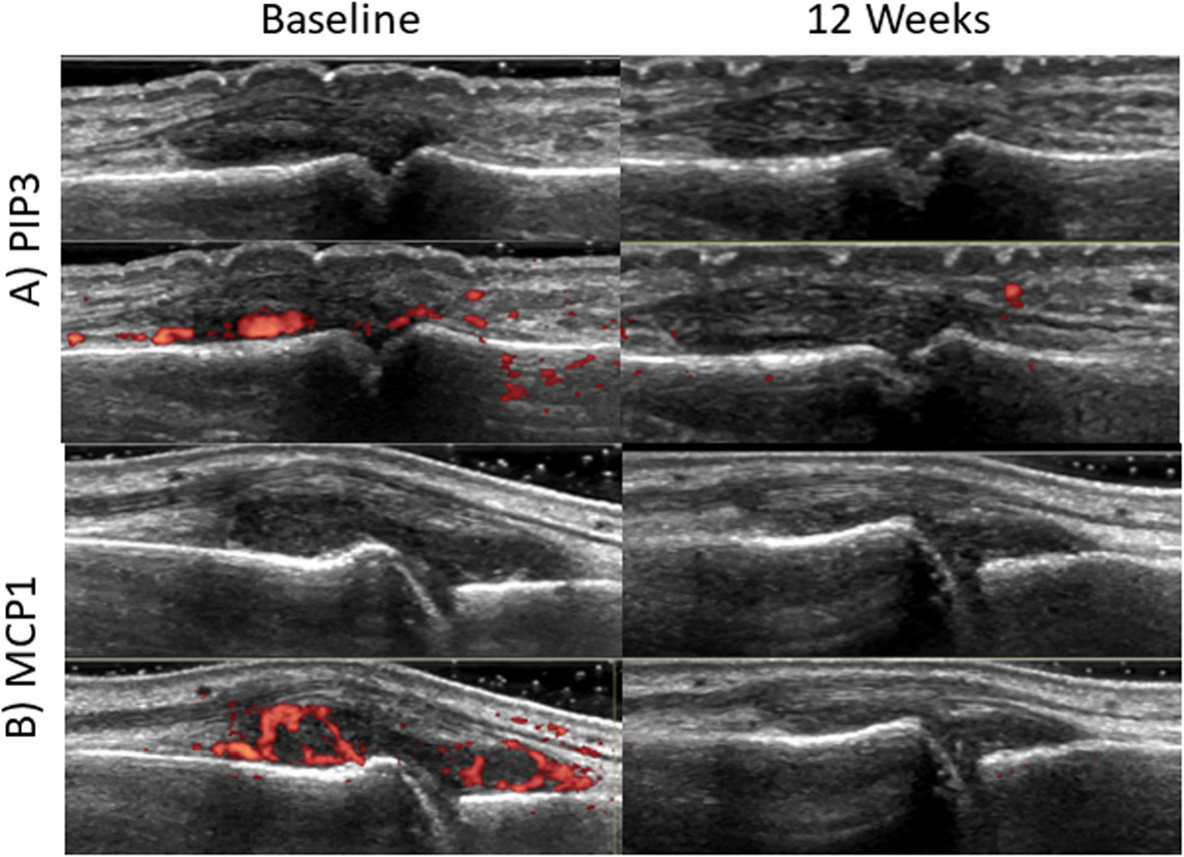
Baseline and 12-Week MSUS images after treatment with tofacitinib (GSUS and PDUS). Panels a and b show images of PIP3 and MCP1 in both B-mode and power Doppler before and after treatment with tofacitinib
